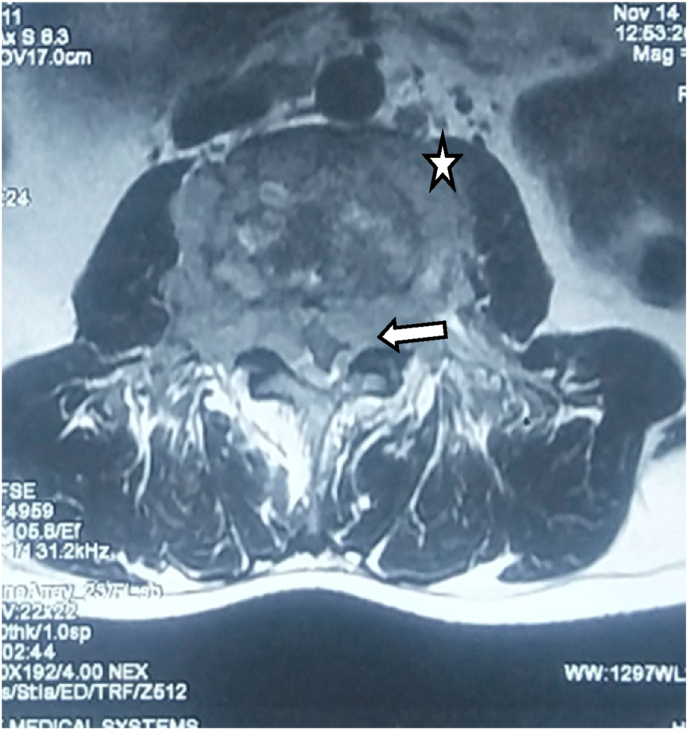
MRI axial T2 image shows circumferential epidural soft tissue lesion (white arrow) causing significant central spinal canal stenosis; Prevertebral as well as paravertebral soft tissue components are also seen (asterisk).
